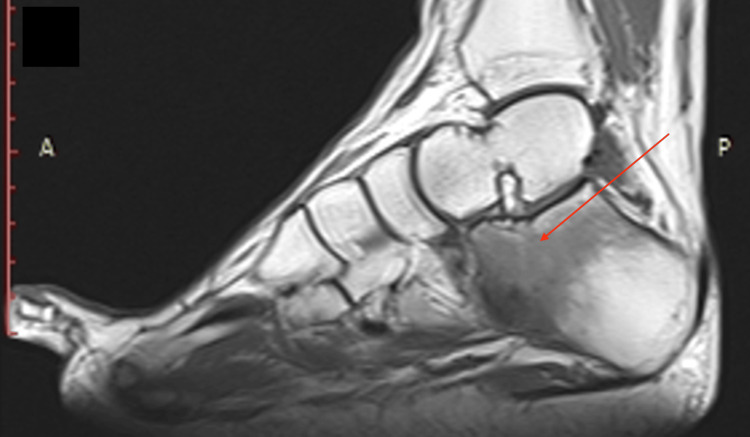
Sagittal view of T1-weighted magnetic resonance imaging (MRI) of calcaneus The red arrow indicates a small, rounded, well-defined nidus and diffuse bone marrow oedema (low signal - dark) in the affected anterior, superior, and middle regions of the calcaneus.
